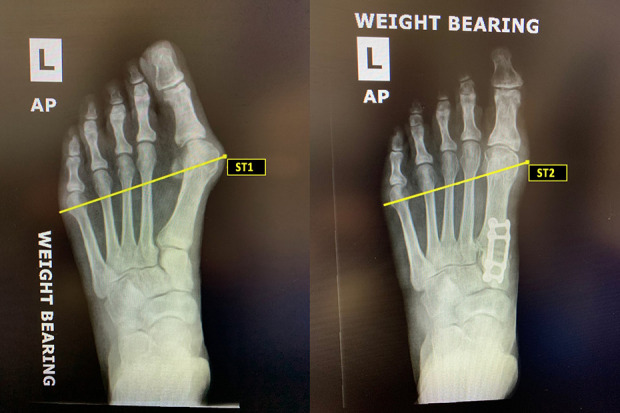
Weightbearing radiographs showing changes in soft tissue foot width preoperative (ST1) and postoperative (ST2) after triplanar first tarsometatarsal arthrodesis. Soft tissue width was defined as the distance from the most medial soft tissue overlying the first metatarsal head to the most lateral soft tissue overlying the fifth metatarsal head.
